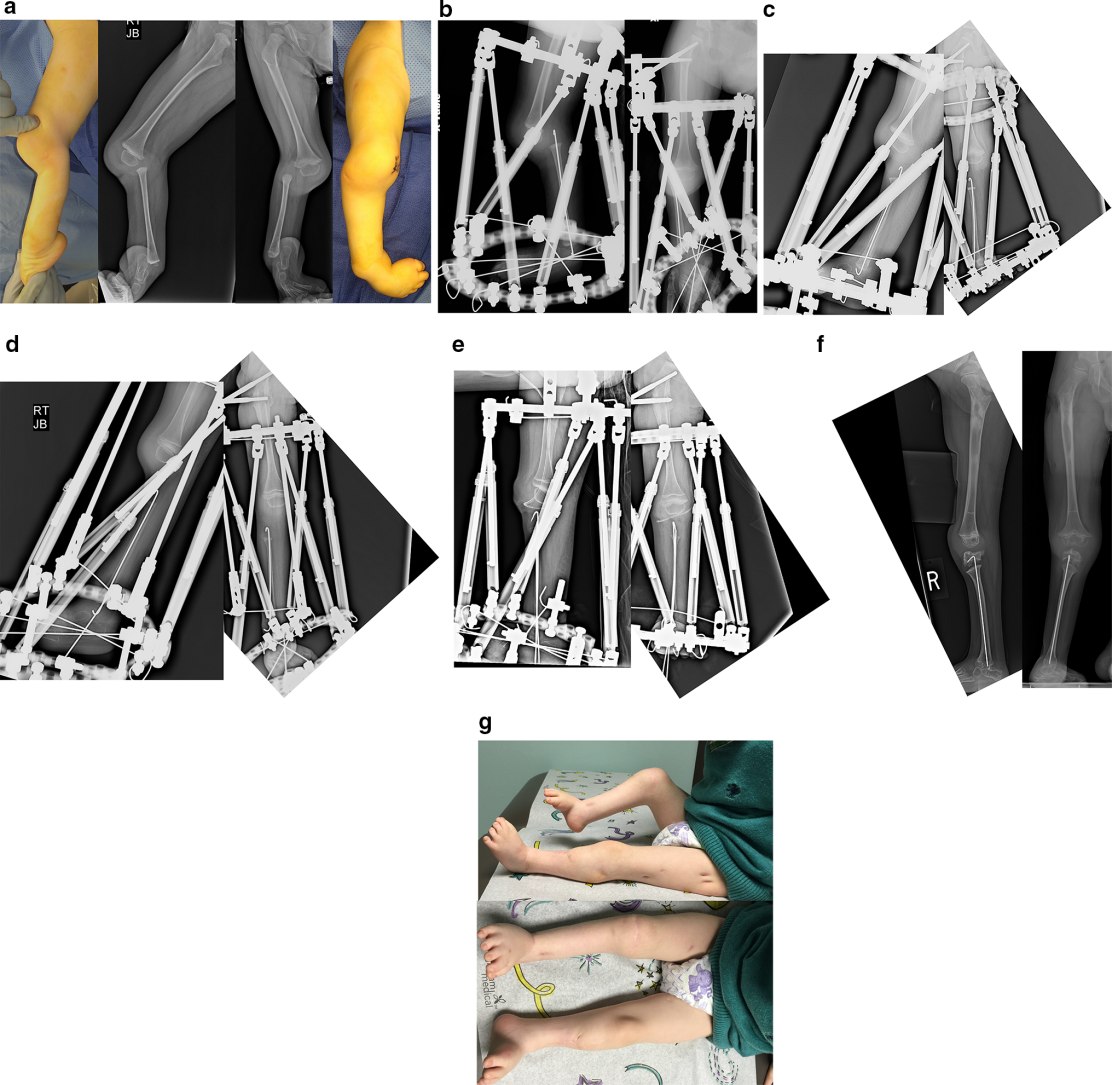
a Lateral (*left* and *center left*) and AP (*right* and *center right*) photographs and radiographs of the right leg of a 2-year-old boy with bilateral tibial hemimelia. Only the *right* side is shown. The *right* side has Paley type 5A tibial hemimelia and the *left* side Paley type 4A. Note the flexion contracture of the right knee and the equino-varus-adductus foot deformity. b Lateral (*left*) and AP (*right*) radiographs showing the TSF in place with femoral and foot rings. The fibula is secured to the foot ring with a transverse wire. Note the temporary epiphysiodesis wire in the fibula hooked at both ends. Also note the wire across the neck of the talus. c Lateral (*left*) and AP (*right*) radiographs at the end of gradual distraction with TSF. The fibular head is centered under the end of the femur. The foot position has not changed and the transverse fibular wires remains connected to the foot ring. d Lateral (*left*) and AP (*right*) radiographs showing that the transverse fibular wire was fixed to the proximal ring using a threaded rod and post. This wire was released from the distal ring to allow gradual correction of the equino-varus foot contracture and to bring the talus under the distal fibula. A second computer planning is carried out to generate a new adjustment schedule for the patient. Note that there is no ossification of the proximal fibular epiphysis. Also note the growth lines related to preoperative infusion of zolidronic acid to prevent disuse osteoporosis during distraction. e Intraoperative lateral (*left*) and AP (*right*) radiographs after the surgery to perform a patellar arthroplasty and fibula–talar fusion is completed. Note the hemovac drain can be seen at the knee. The Paley–Weber patellar arthroplasty was performed fixing the proximal fibular epiphysis to the patella with a hooked intramedullary wire secured to the bottom of the frame below the foot. The talus was fused to the distal tibial epiphysis. BMP2 was inserted into a drill hole in the patella and proximal fibula to lead to ossification and fusion of both the patella and fibular epiphysis. A transverse distal fibular wire is arced to the foot ring to apply compression across the fusion site. f Lateral (*left*) and AP (*right*) radiographs showing the patella and proximal fibular epiphysis are ossified and fused together with preservation of the proximal fibula physis. The patella now serves as a tibial plateau. The talus has also fused to the distal fibula but the distal fibular physis has closed. g Clinical photographs, frontal view (*bottom*) and medial view (*top*) showing that the right knee can bend to 90° (*top*). There is active motion present. The other leg was also successfully treated for type 4A tibial hemimelia
